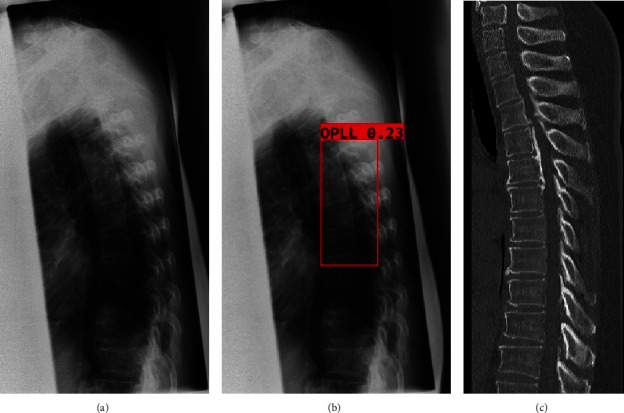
Object detection method: (a) plain lateral radiographs of the thoracic spine; (b) final region with the highest probability; (c) sagittal plane CT image to identify OPLL. CT: computed tomography; OPLL: ossification of the posterior longitudinal ligament.
